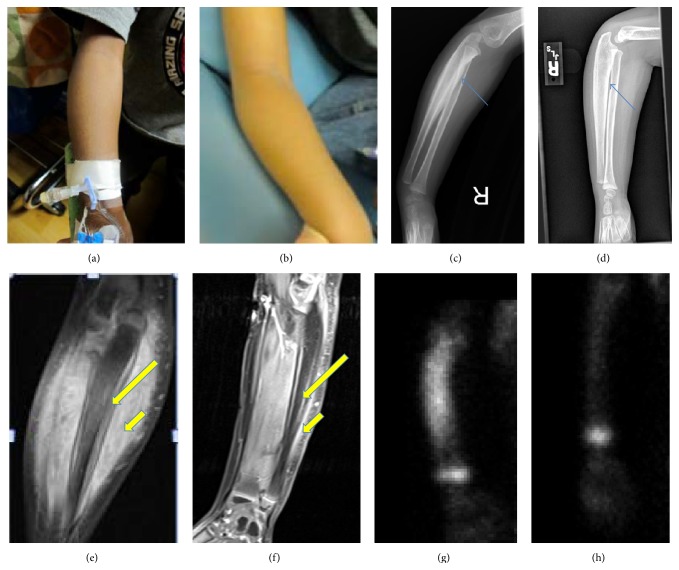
Comparison of right arm before and after treatment with exam and images. (a) Right arm before treatment. (b) Right arm after treatment showing resolution of right forearm hypertrophy. (c) Right arm X-ray before treatment. (d) Right arm X-ray after treatment: follow-up radiographs revealed near total resolution of the periosteal reaction (cf. thin blue arrows). (e) Right arm MRI before treatment. (f) Right arm MRI after treatment: follow-up MRI of the right forearm revealed resolution of periostitis (cf. long yellow arrows) and myositis (cf. short yellow arrows). (g) Right arm bone scan before treatment. (h) Right arm bone scan after treatment: follow-up revealed total resolution of the abnormal uptake in the forearm.
